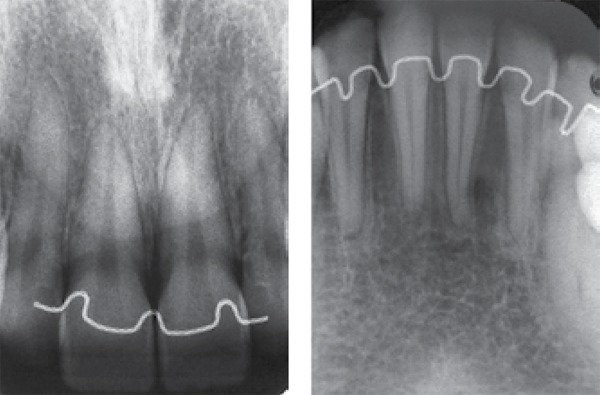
Final periapical radiographs of maxillary and mandibular incisors.
